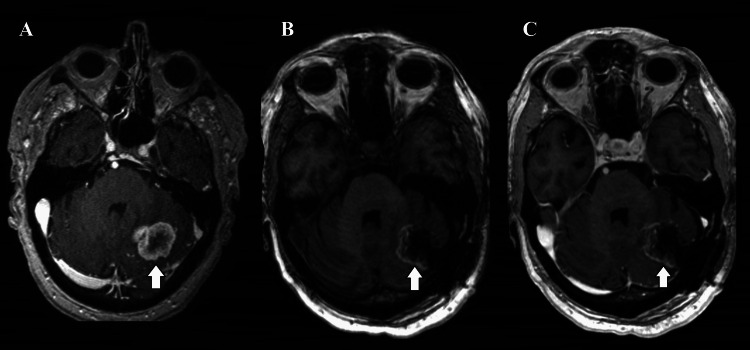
Preoperative T1-weighted MRI demonstrating the brain metastasis (A, arrow). T1-weighted MRI demonstrating resection without (B) and with (C) contrast (arrows). MRI: magnetic resonance imaging
